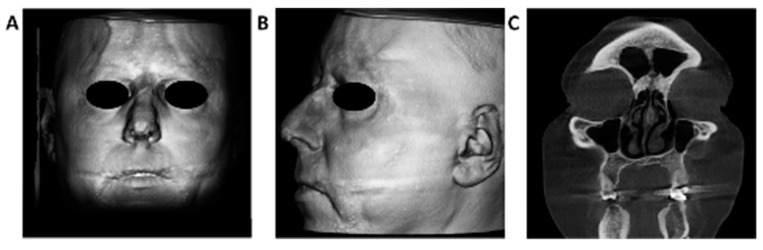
Cone-beam CT. 3D facial reconstruction showing papulonodular lesions on the forehead, nose, cheeks, upper lip, chin (A,B); Inflammatory changes at the level of the ethmoidal mucosa on the right side (C).
